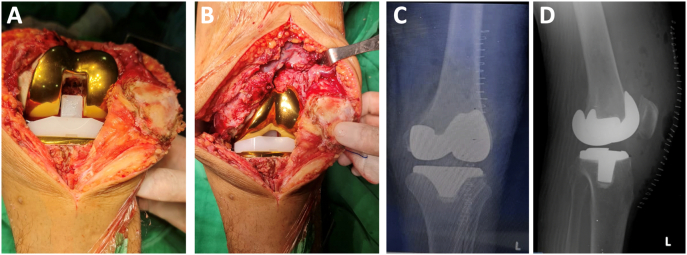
Intraoperative photograph after insertion Unconstrained PS Design Knee Implant (A)(B), Post-operative Xray Anteroposterior Lateral View (C)(D).
